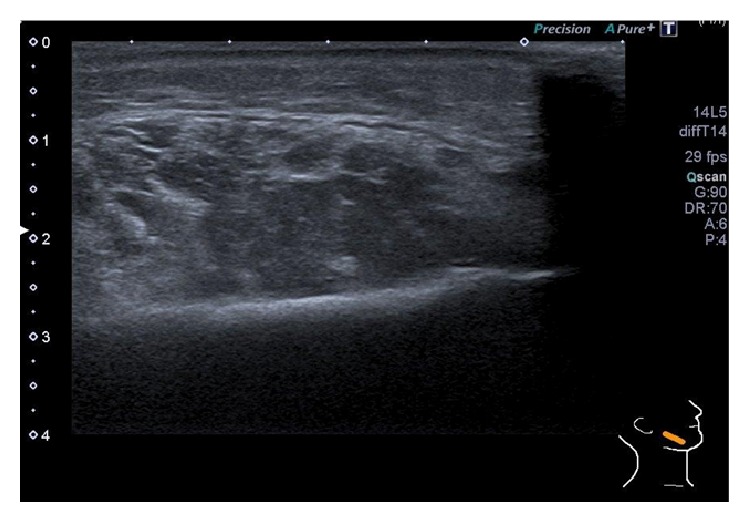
Ultrasound scan of the face shows a subcutaneous haematoma around the right masseter muscle measuring 2 cm in depth. No other pathology was found.
